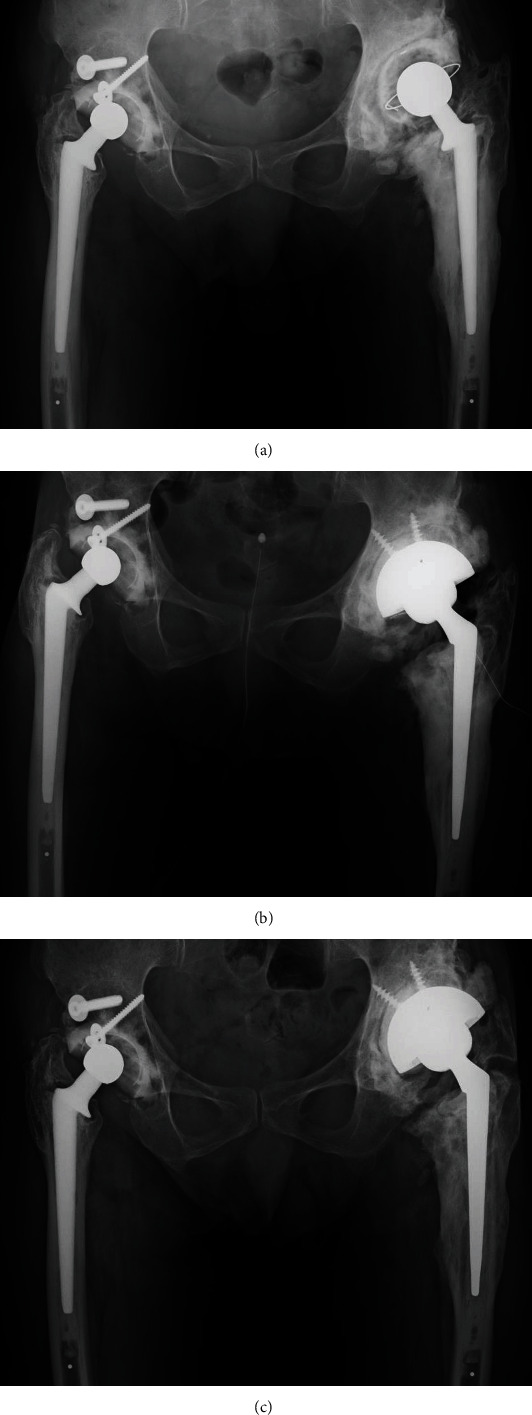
(a) Plain radiograph of a 78-year-old man obtained prior to the third THA, showing a clear zone around the cemented cup. Abbreviations: THA: total hip arthroplasty. (b) Plain radiograph of a 78-year-old man obtained immediately after the third THA, showing the initial gap between the host bone and the allograft in zones 2 and 3. We used vancomycin-mixed cement and replaced the cup and cemented stem simultaneously via a single-stage exchange. (c) Plain radiograph of a 78-year-old man obtained at the final follow-up (3 years postoperatively) examination with the gap between the host bone and allograft persisting in zone 3, with no evidence of prosthesis loosening.
